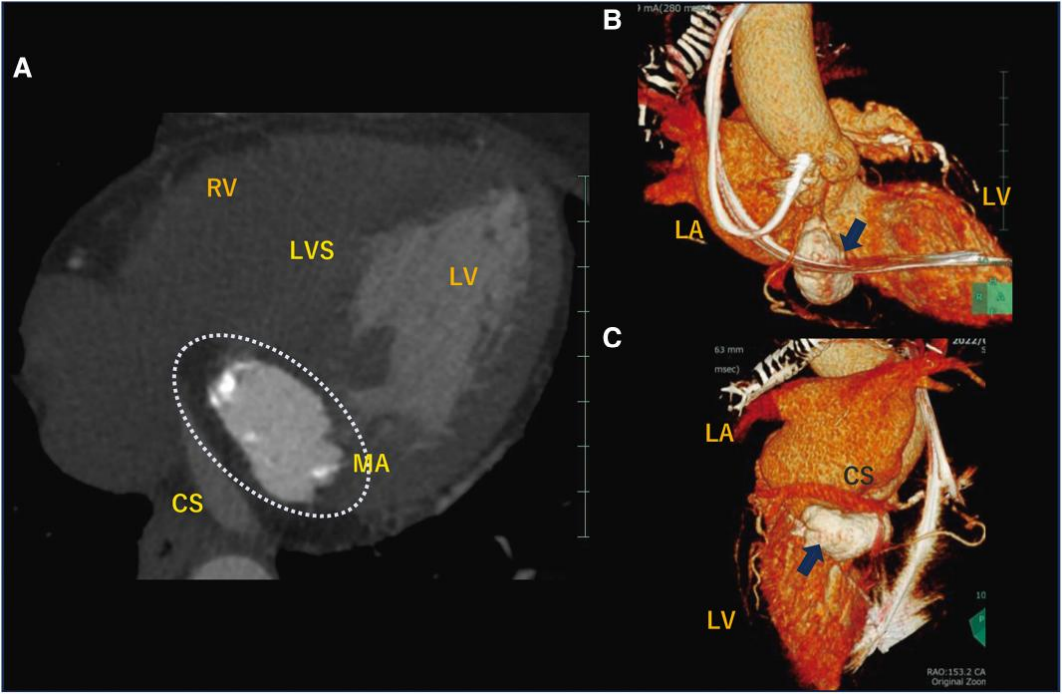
Computed tomography with contrast: the massive calcification of the posterior mitral leaflet (*A*). Three-dimensional volume rendering showing the mass from the right oblique view (*B*) and the posterior view (*C*) (removing the right atrium and ventricle). LA, left atrium; LV, left ventricle; RV, right ventricle; MA, mitral annulus; CS, coronary sinus; LVS, left ventricular septum.
